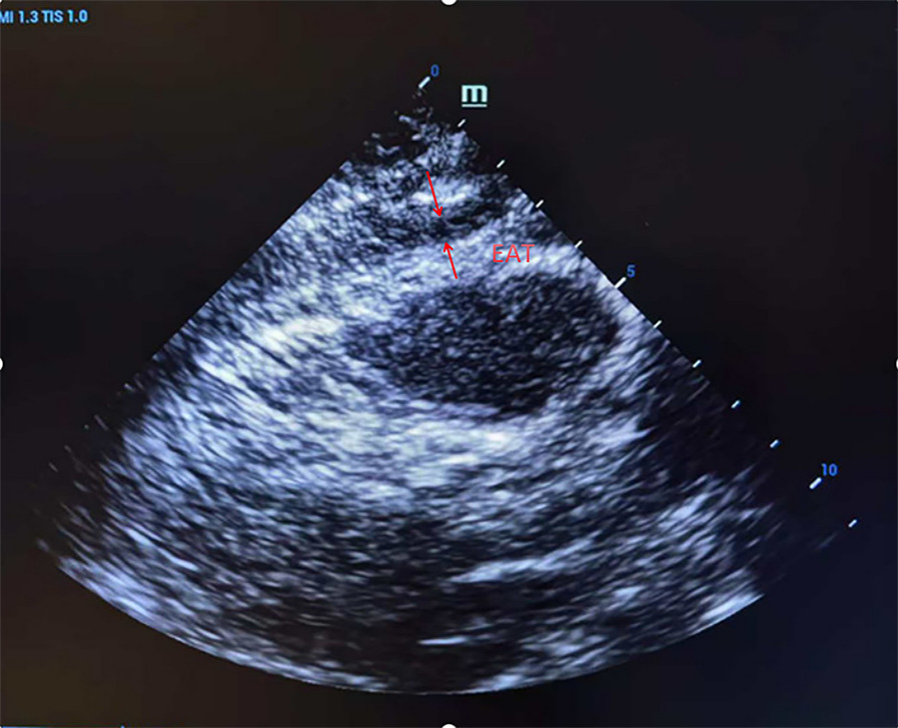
Ultrasound detection of right ventricular anterior epicardial adipose tissue (EAT) thickness in parasternal long-axis view. A representative echocardiographic image showing a measurement of EAT thickness located between the right ventricular free wall and the visceral pericardium, taken at end-diastole.
